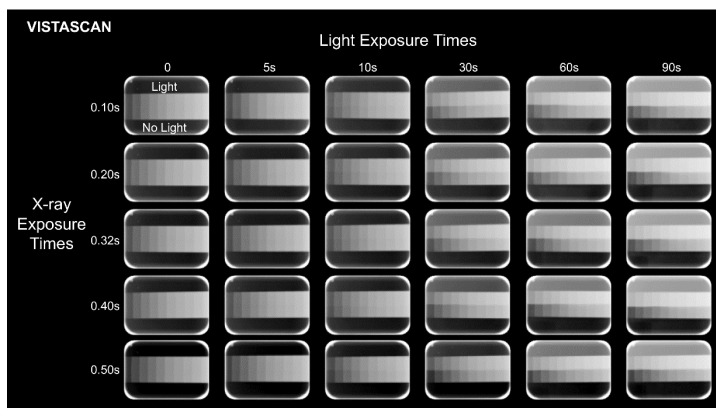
Representative radiographs of each experimental condition obtained using VistaScan. All images are shown using the same display window and level.
